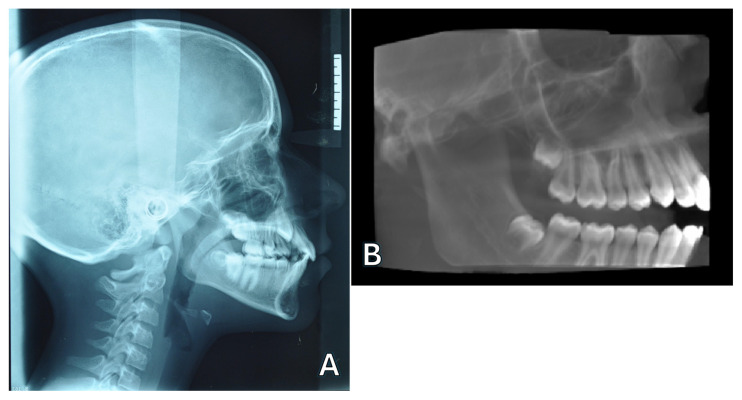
(A) 2D conventional lateral cephalogram and (B) lateral cephalogram obtained from 3D CBCT.
